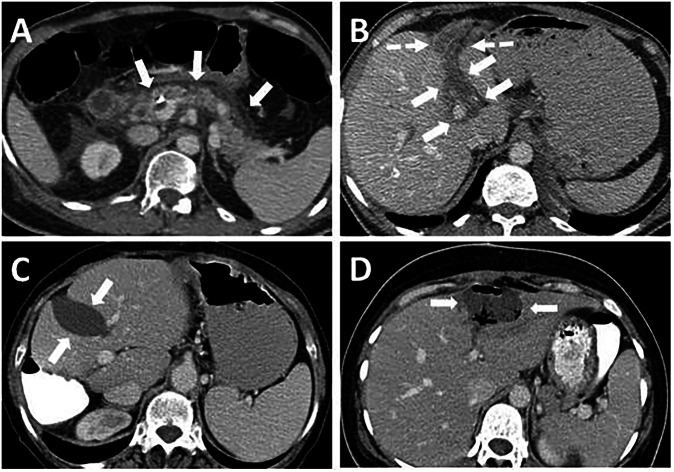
FL and LTH involvement in pancreatitis. A, B 68-year-old male patient presenting with epigastric pain. (A) Axial plane contrast-enhanced CT image shows peripancreatic fat stranding (arrows) compatible with acute pancreatitis. B Inflammations of the FL (dashed arrows) and LTH (arrows) are also present. C 50-year-old male patient with a history of right hepatectomy for metastatic colorectal cancer two years ago. He also had a history of acute pancreatitis 6 weeks ago and now presents with right upper quadrant pain. The axial plane CT image shows a fluid collection in the FL (arrows). The lesion was considered to be a pseudocyst. Follow-up imaging study demonstrated the decrease in the lesion size (not shown). D 68-year-old male patient with a history of acute pancreatitis and FL abscess. Axial plane contrast-enhanced CT image shows a fluid collection with air-fluid levels in the FL (arrows)
